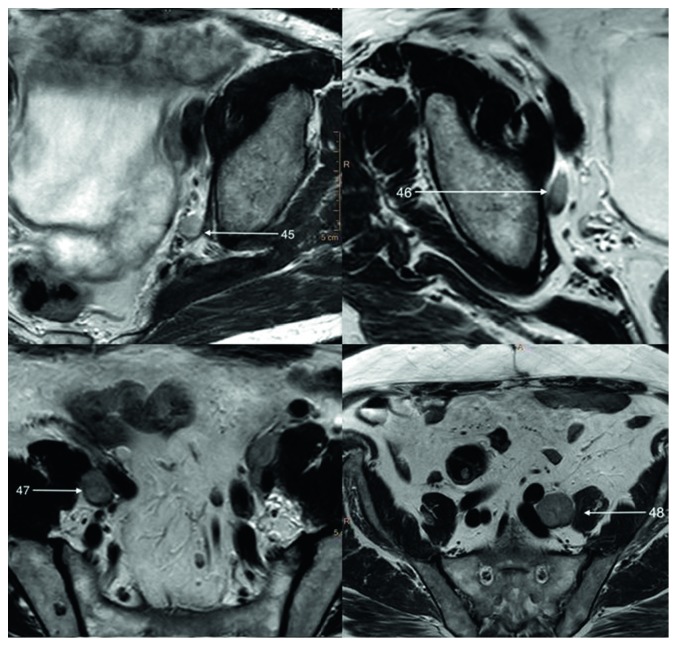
T2-weighted axial image of enlarged regional prostatic lymph nodes.
